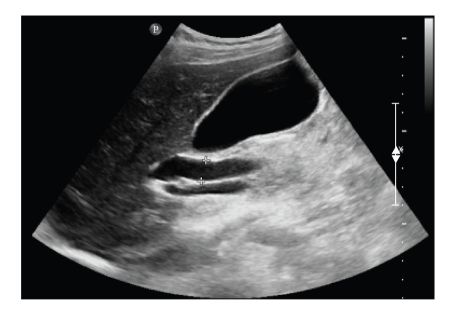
Abdominal ultrasound showing a dilated common bile duct (11 mm).
